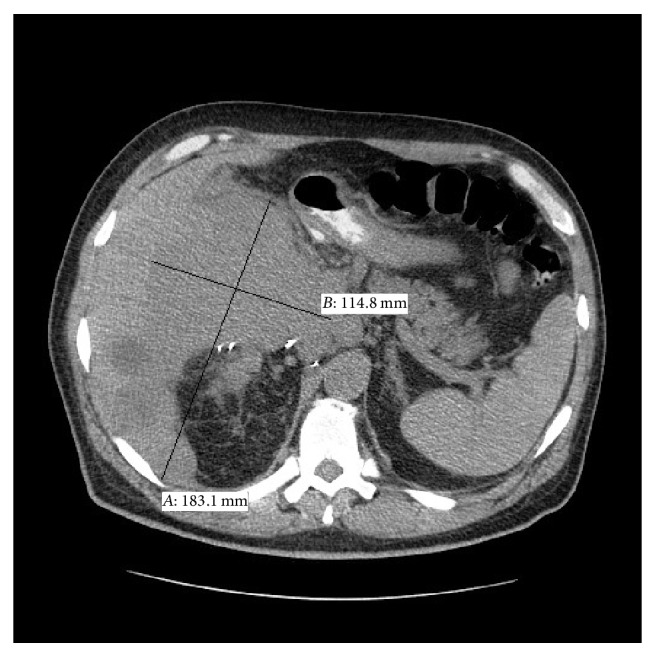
CT image showing involvement of kidney and liver by the tumor.
